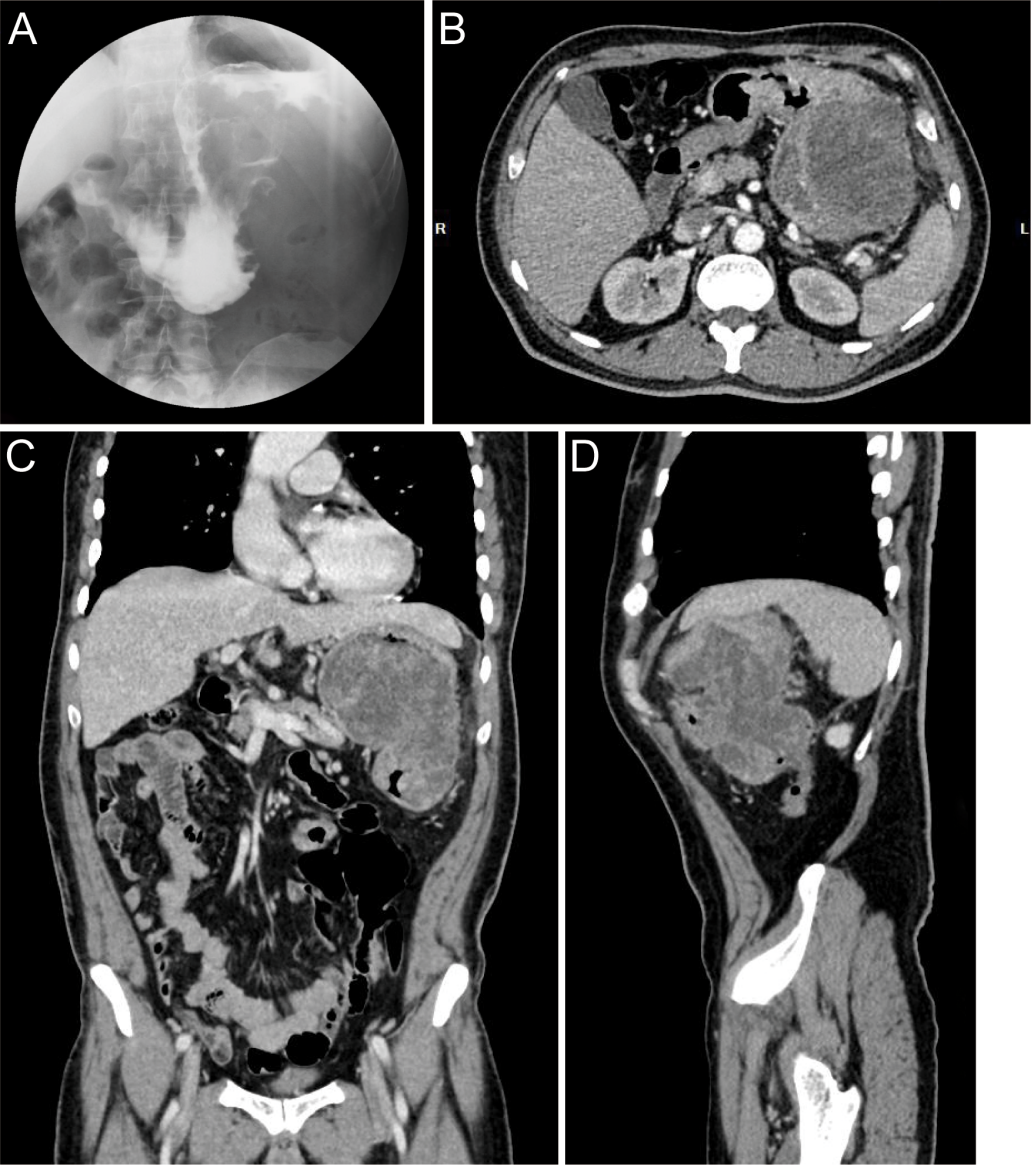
Preoperative radiographic images. (A) Upper GI series with barium contrast showing a large filling defect in the greater curvature gastric wall. Axial (B), coronal (C), and sagittal (D) contrast-enhanced CT images documenting a large mass contiguous with the gastric body.
